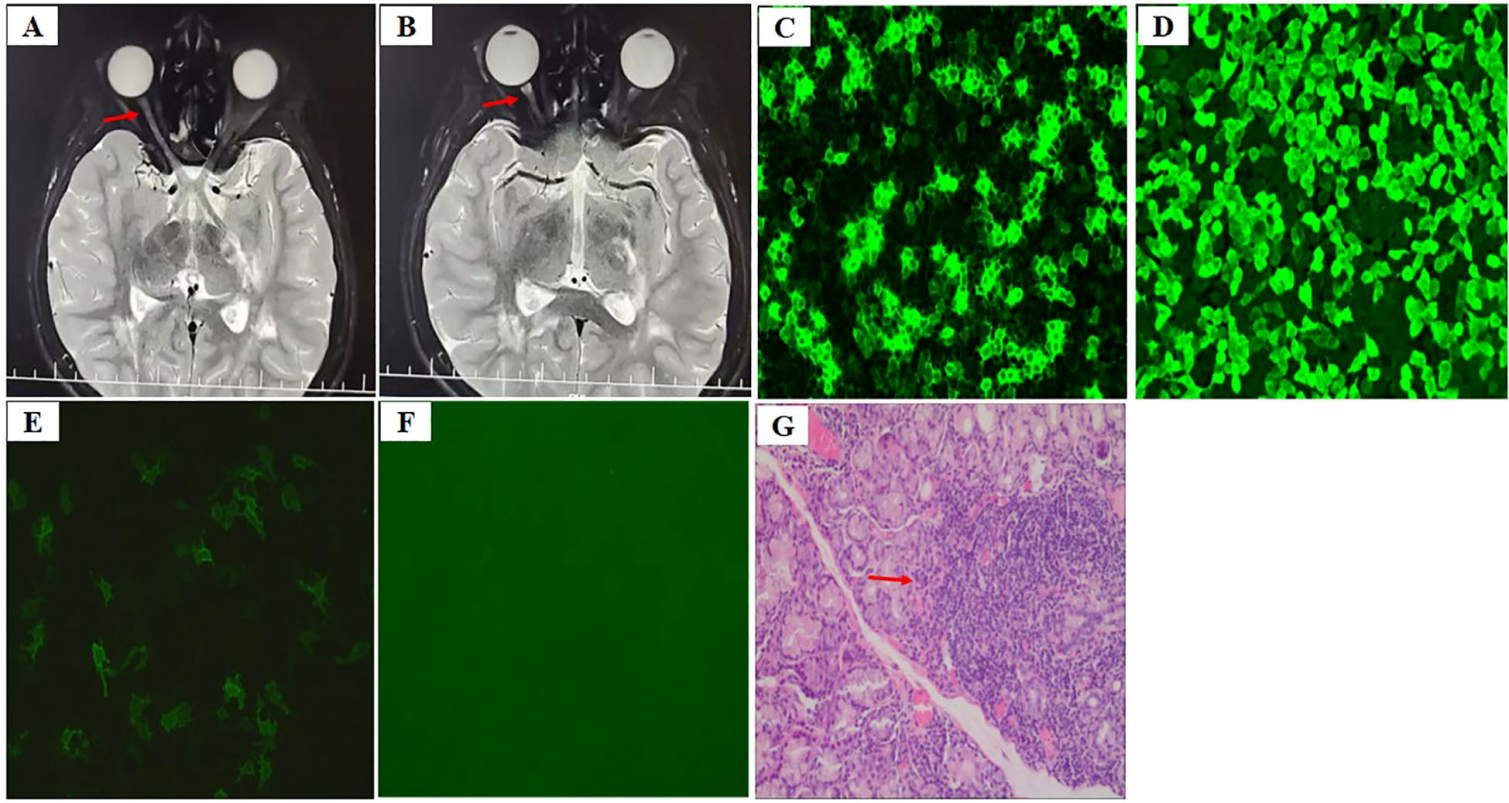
(A, B) Axial T2-weighted image shows thickening of the right optic nerve (A) and hyperintense lesion in the optic nerve sheath (B) [arrows in (A, B)], (C) AQP4-IgG in the serum at an AQP4 titer of 1:1000 by cell-based assay (CBA) before therapy, (D) AQP4-IgG for positive control, (E) AQP4-IgG in the serum at an AQP4 titer of 1:100 during preventive therapy, (F) AQP4-IgG for negative control, (G) Labial salivary gland biopsy displays focal lymphocytic sialadenitis with 1 focus/4 mm2 [arrow in (G)].
